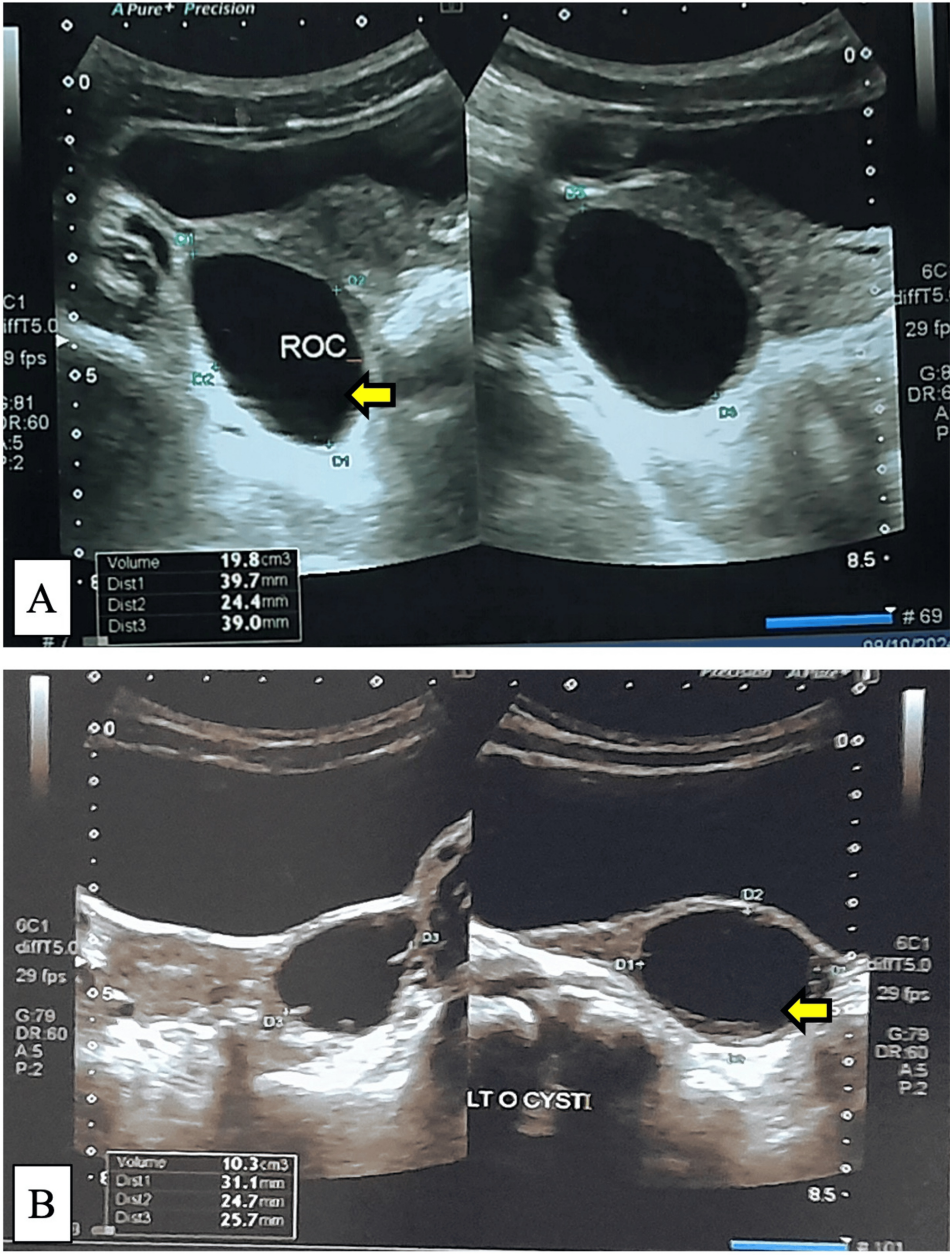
Ovarian cyst on pelvic ultrasound. A. 5.7-year-old girl presenting with breast budding; pelvic ultrasound shows a right ovarian cyst measuring 3.9 × 2.4 × 3.9 cm. B. 4.6-year-old girl presenting with stage 2 breast enlargement and menarche; pelvic ultrasound shows a left ovarian cyst measuring 3.1 × 2.6 × 2.5 cm.
